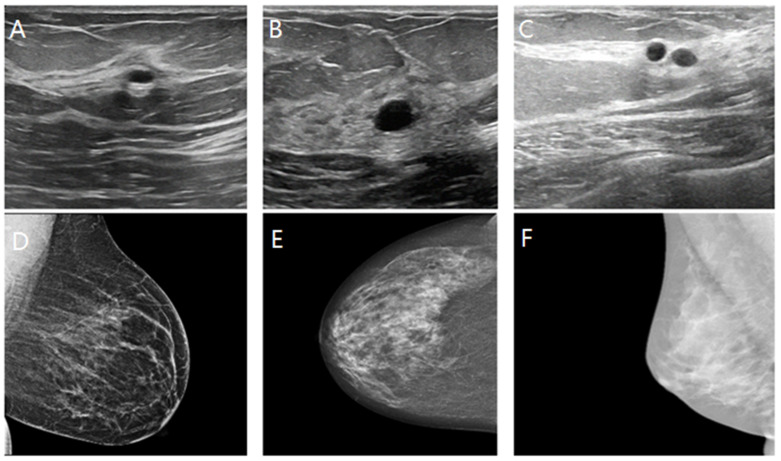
Comparison of breast tissue textures in sonography and mammography images.
